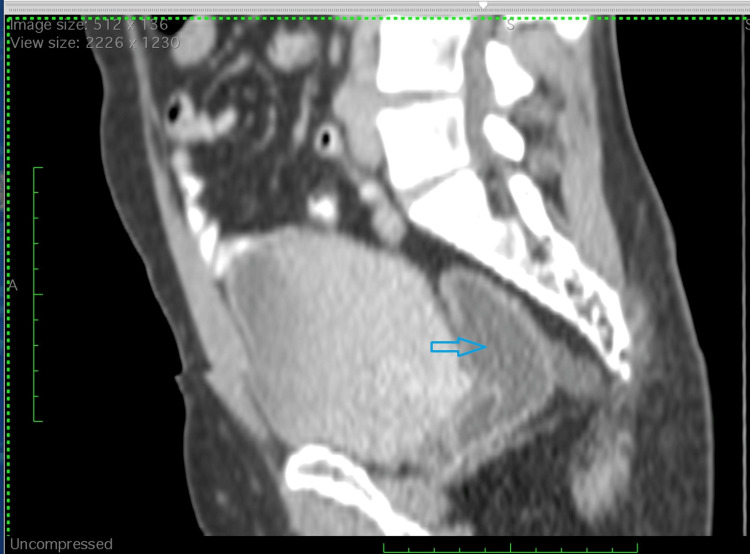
Contrast-enhanced computed tomography (CECT). Contrast-enhanced computed tomography (CECT) of the sagittal section of pelvis showing well defined, tubular peripherally enhancing lesion in the rectovesical space marked by arrow extending inferiorly toward the anal verge. The lesion demonstrates homogeneous fluid attenuation without definite solid components or septations. No communication with the bladder, prostate, or rectum is evident.
